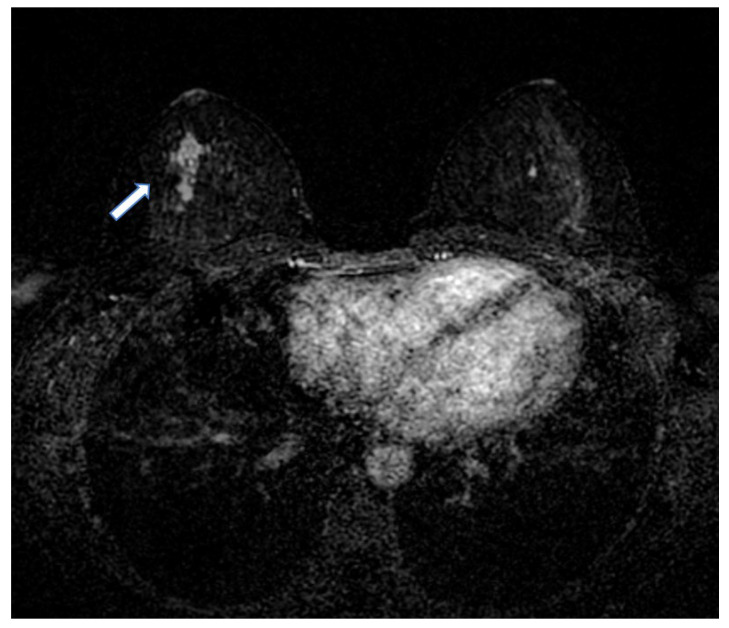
Breast MRI shows an enhancement (arrow) in the right breast suspicious for breast cancer.
